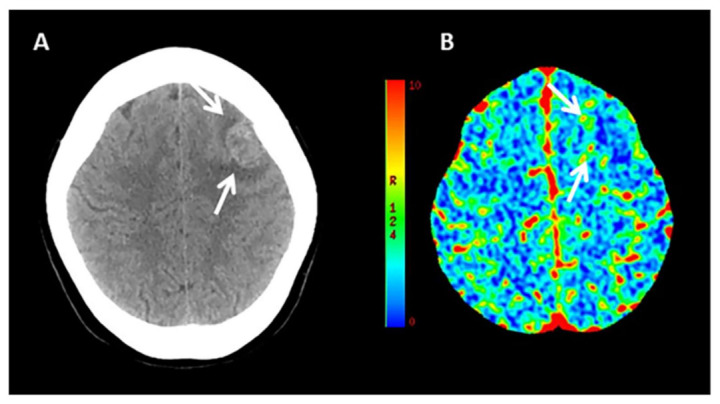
Example of CT Perfusion in a patient with ICH due to malignancy. Non-contrast CT (NCCT) scan (A) and cerebral blood volume (CBV) map (B) in a patient with acute ICH due to the bleeding of an underlying high grade glioma located left frontal lobe and characterized by elevated CBV values in the perihematomal region (arrows).
